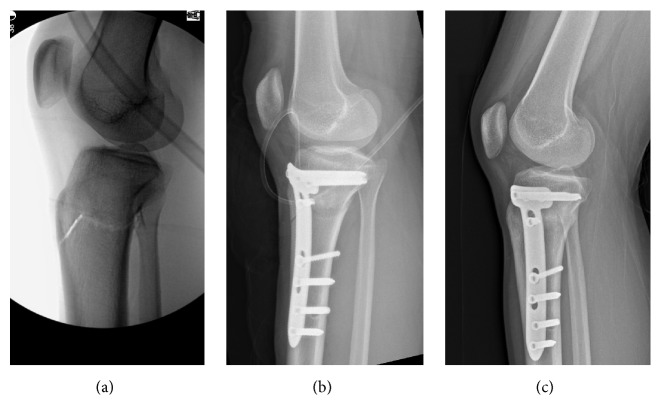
Lateral radiographs of open wedge high tibial osteotomy with distal tubercle osteotomy: (a) biplanar retrotubercle osteotomy (intraoperative view); (b) locking plate fixation at osteotomy site (immediately postoperative); and (c) union of the osteotomy site (postoperative month 3).
